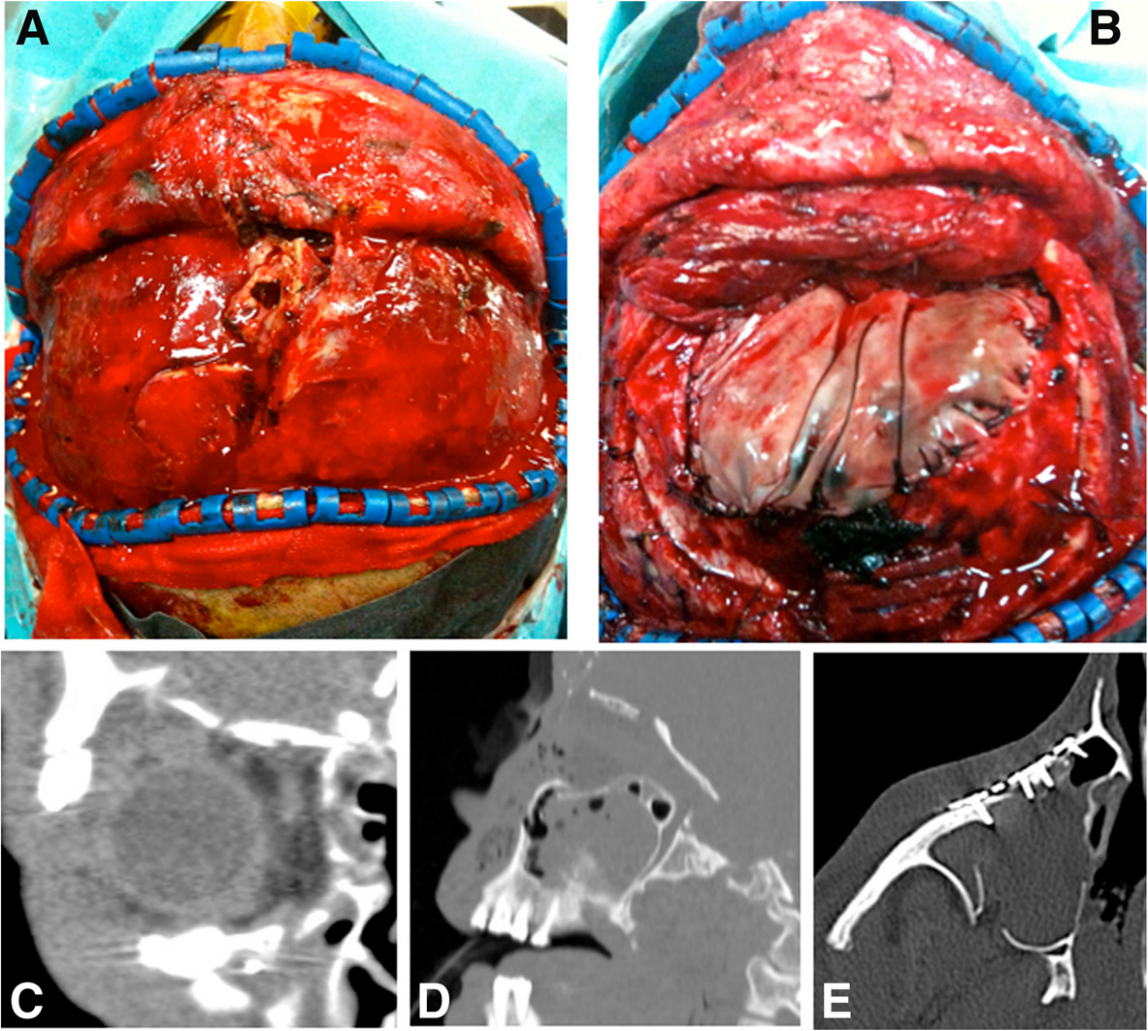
Skull and maxilla reconstruction: surgical and radiological view. A: Intraoperative view at the opening, with fracture of frontal bone and necrotic brain through the bone gap. B: Intraoperative view after duroplasty and cranialisation of the frontal sinus by galeal flap. C, D and E: CT scan, coronal, sagittal and axial scans, demonstrating the reconstruction of the orbit floor and roof and zygoma.
